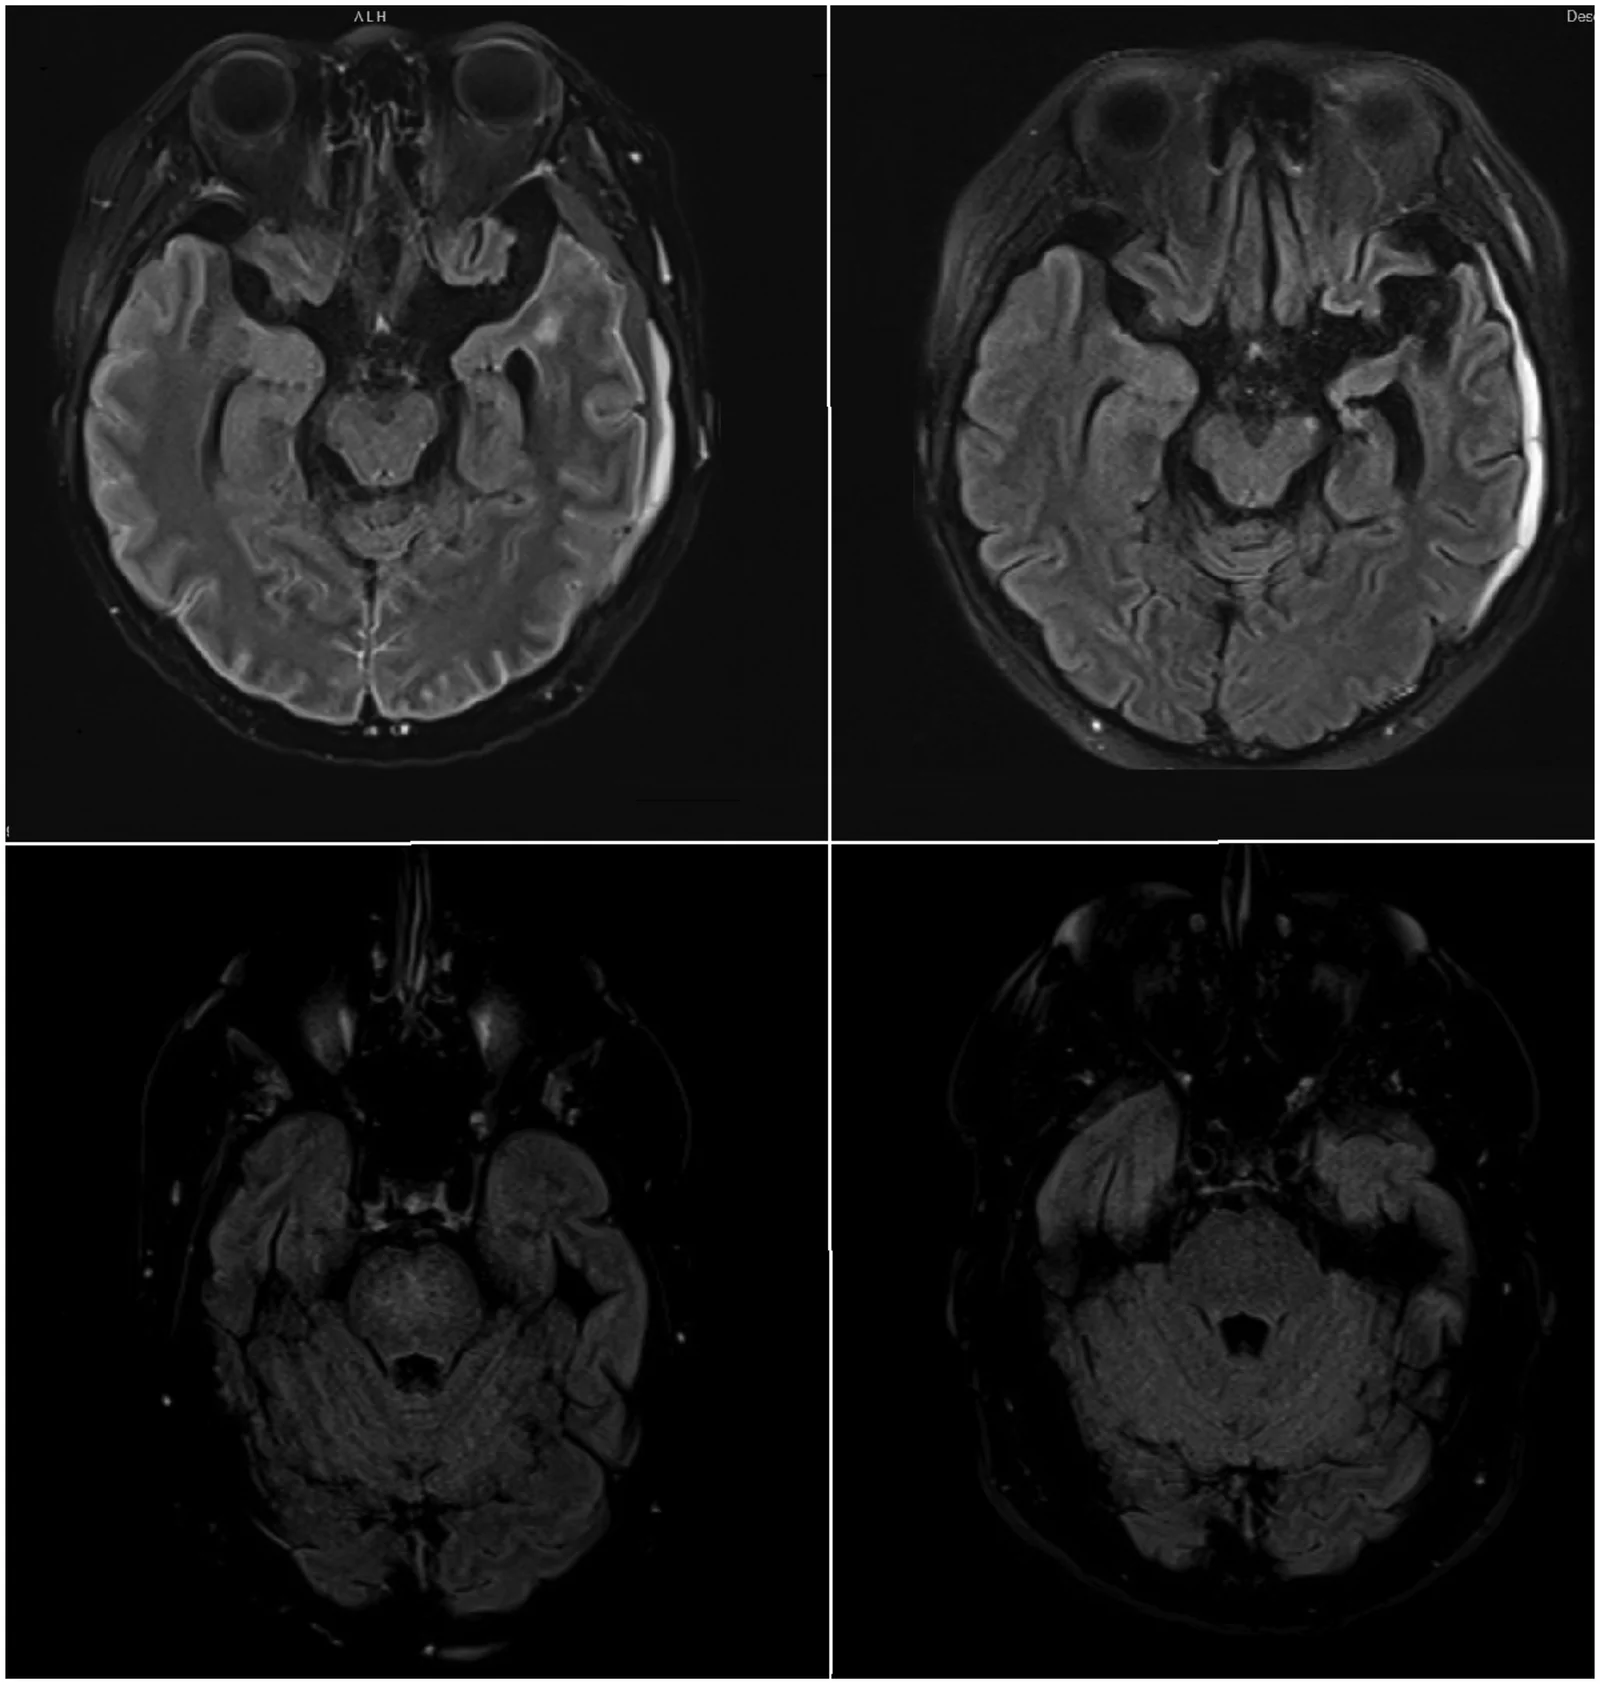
Top: MRI T2/FLAIR sequence showing the diffuse T2 leptomeningeal hyperintensity (left image 30 days post-CAR-T and right pre-CAR-T). Bottom: MRI T2/FLAIR sequence showing the progressive T2 brainstem hyperintensity (left post-CAR-T, right pre-CAR-T).
